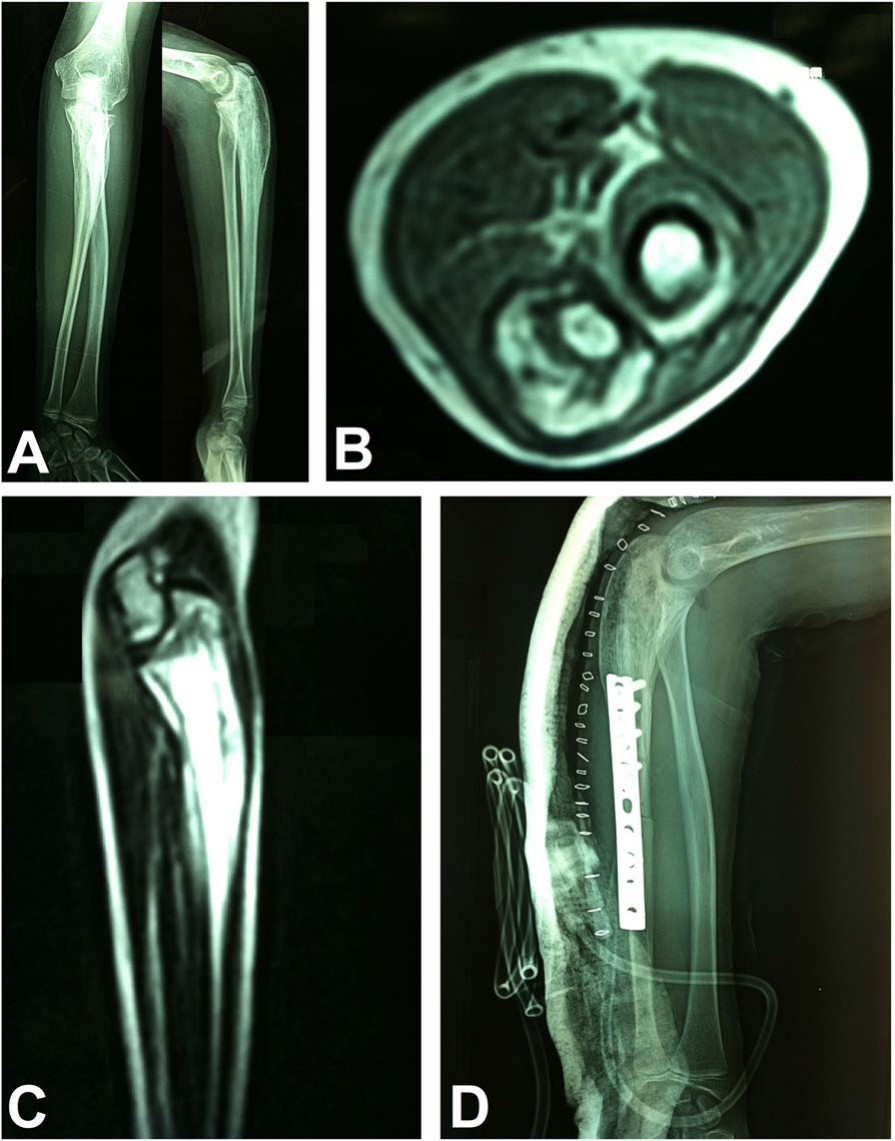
A 12-year-old female with Ewing's sarcoma of the proximal ulna treated by resection, extracorporeal freezing and reimplantation with plate fixation. A Preoperative X-rays anteroposterior and lateral views. B Preoperative MRI axial view. C Preoperative MRI sagittal view. D Postoperative X-ray lateral view
